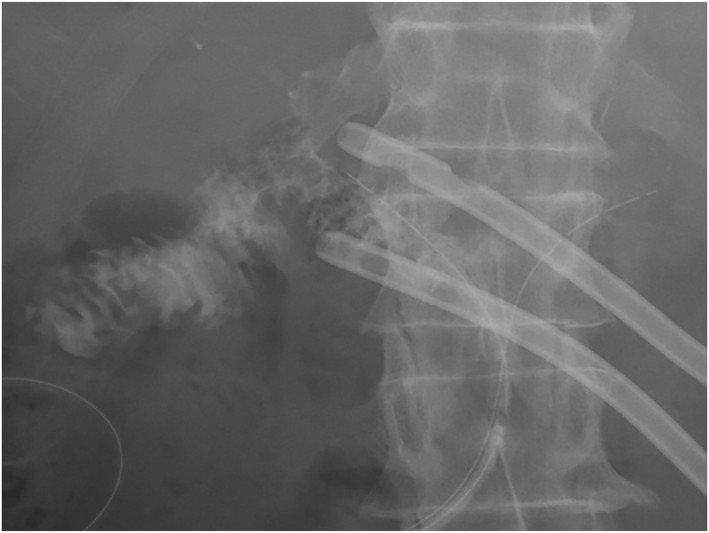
Radiographic image showing drainage into the jejunum.
